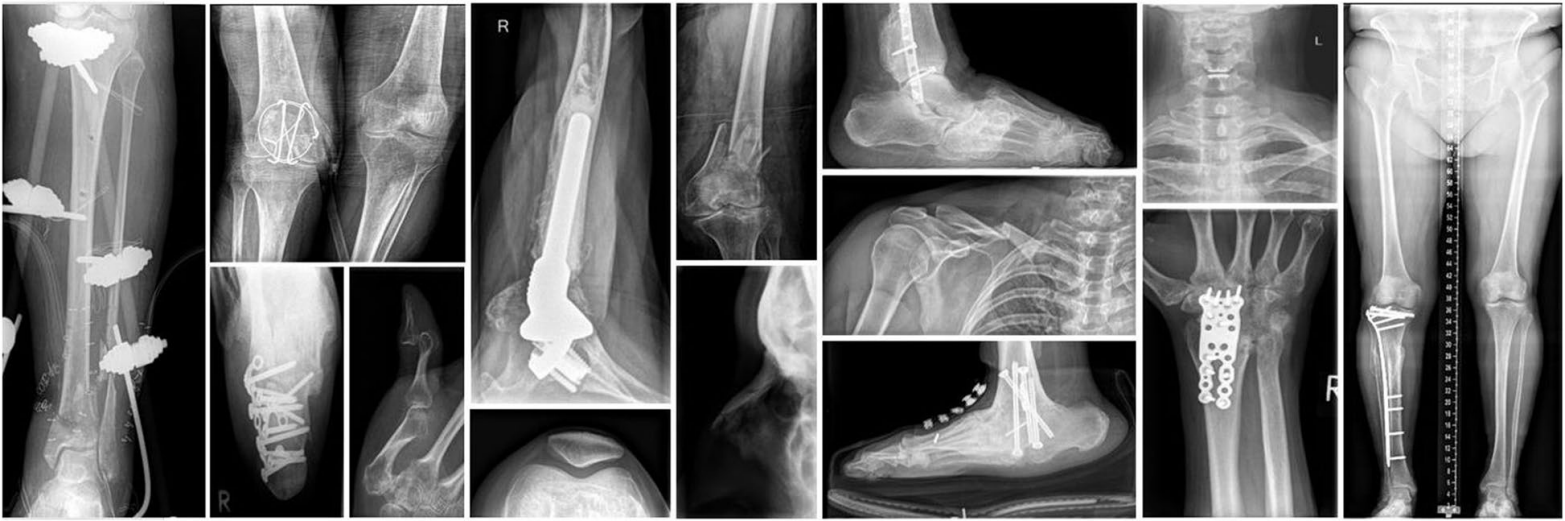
Exemplary cases of the dataset representing the broad variability of body parts, radiographic projections, and pathologies
